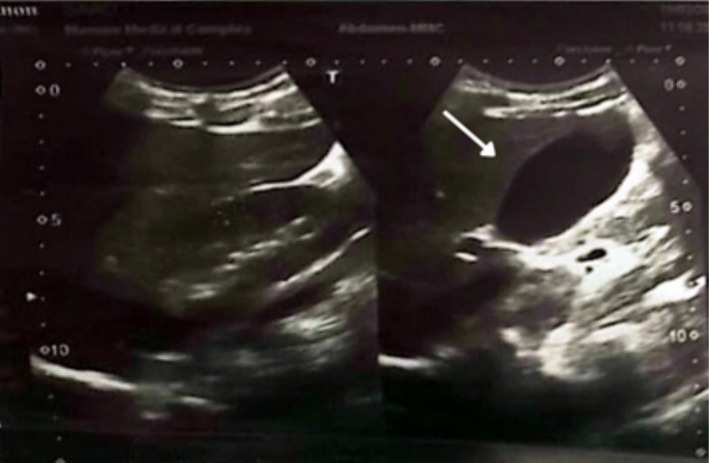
Ultrasound for the whole abdomen: A cystic area measuring 4.0 × 1.3 cm in the pancreas arising from the head towards the body, suggestive of peripancreatic fluid collection.
